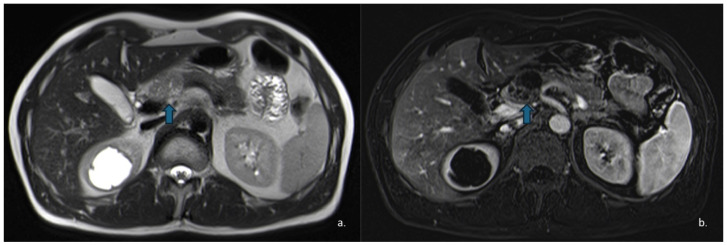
MRI of a 37-year-old woman shows a lesion in the pancreatic head (arrow) with intermediate signal intensity on T2-w HASTE sequences (a) and mild enhancement of the solid component on dynamic arterial-phase sequence (b). Endoscopic ultrasound suggested the diagnosis of a solid pseudopapillary epithelial neoplasm (SPEN).
